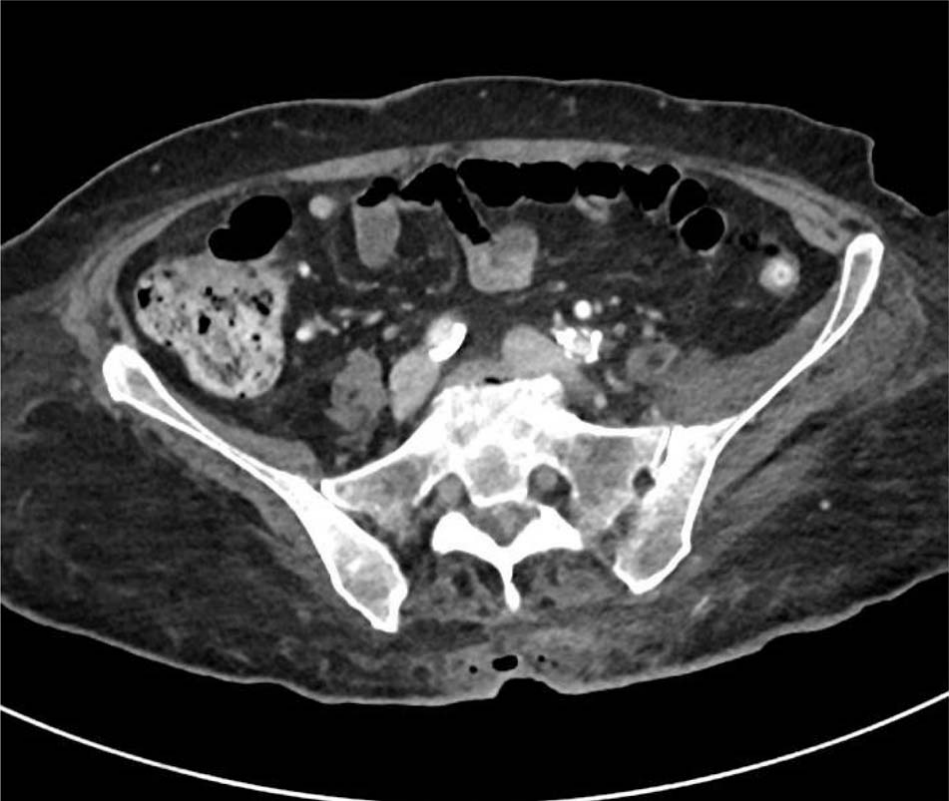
Coronal CT scan image of the pelvis showing fat stranding at the level of the sacrum with air locules.
